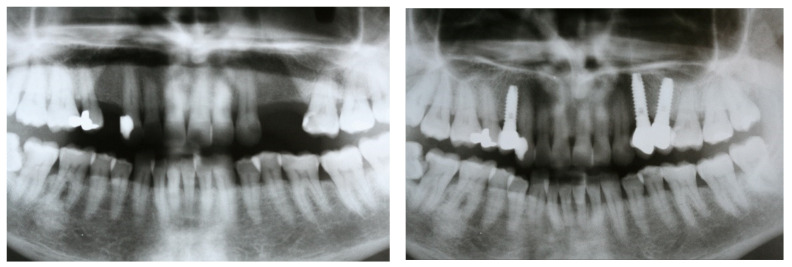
Panoramic radiographs were taken before treatment as part of the diagnosis and treatment plan and after implant placement and prostheses delivery.
